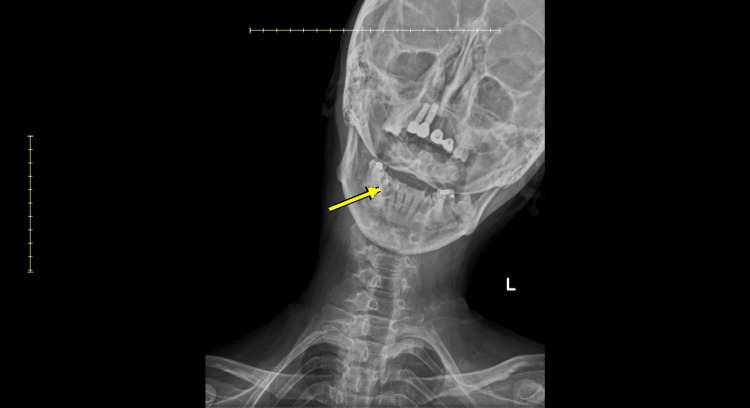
Anteroposterior open-mouth radiograph in left lateral flexion. Arrow indicates the upper cervical region.
